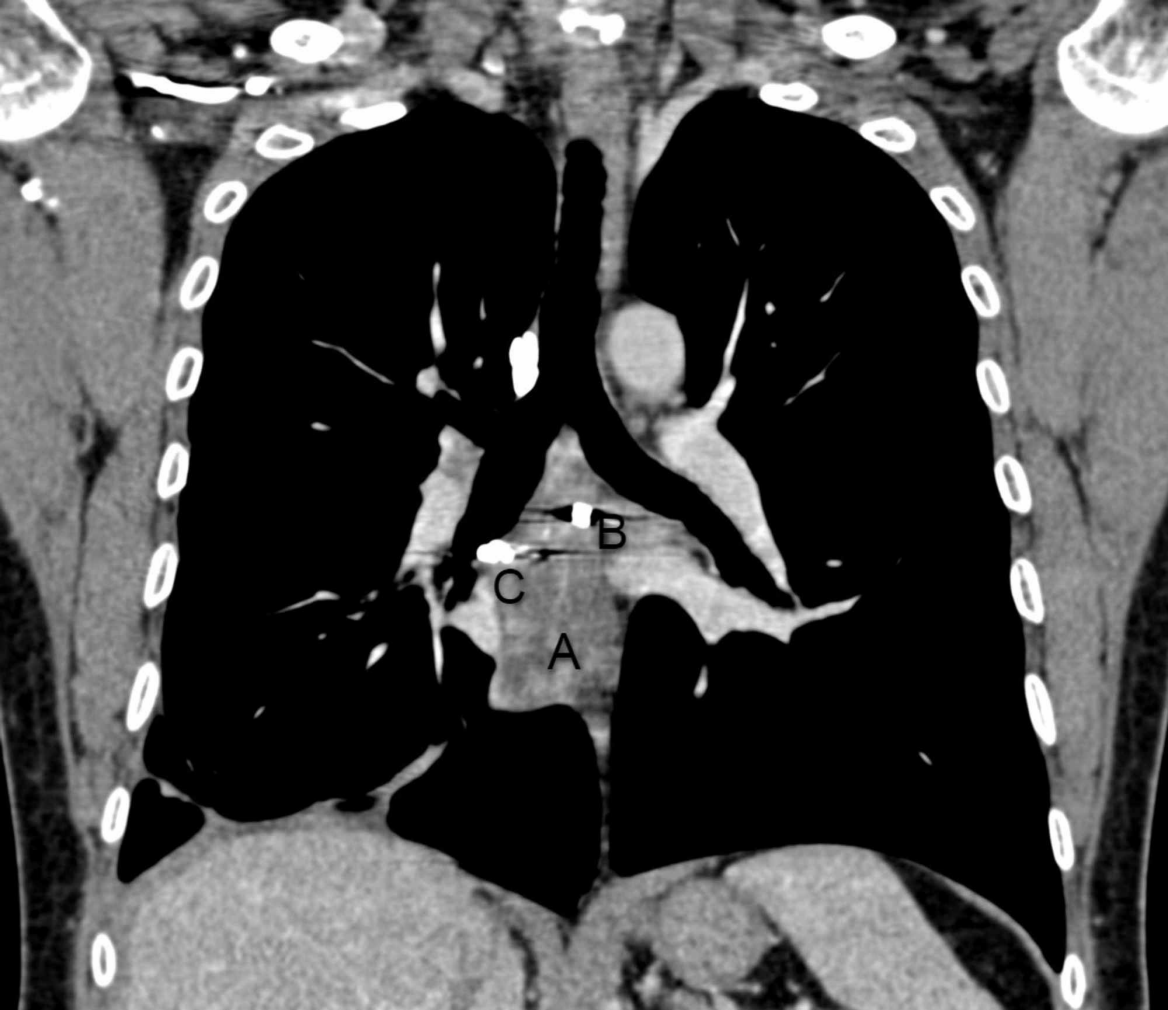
Follow-up coronal CT section after three weeks showing significant interval decrement of mediastinal hematoma with intact mediastinal coils in middle mediastinum. A: mediastinal hematoma B, C: mediastinal coils
